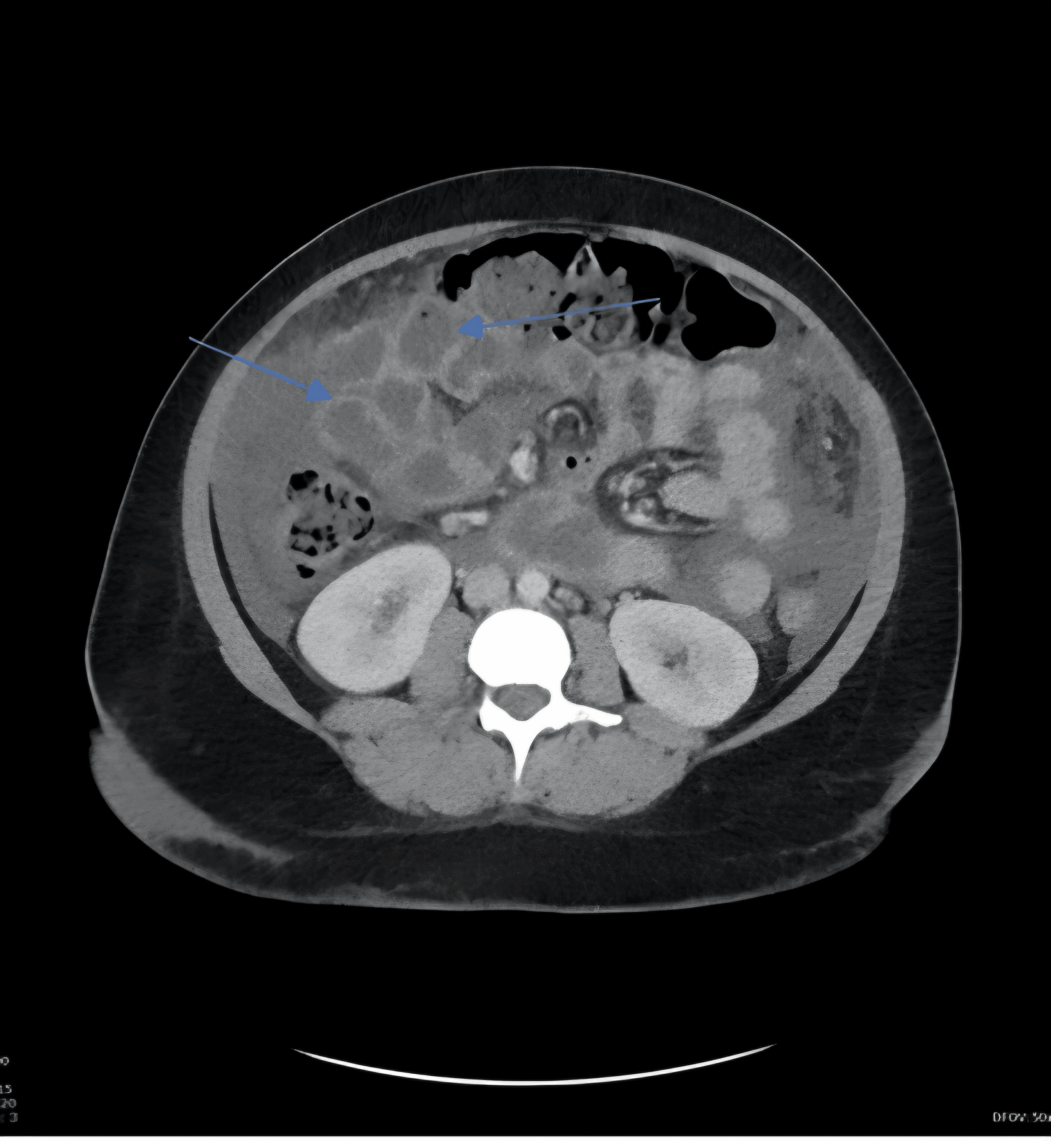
Computed tomography showing mesenteric oedema and peritoneal thickening
